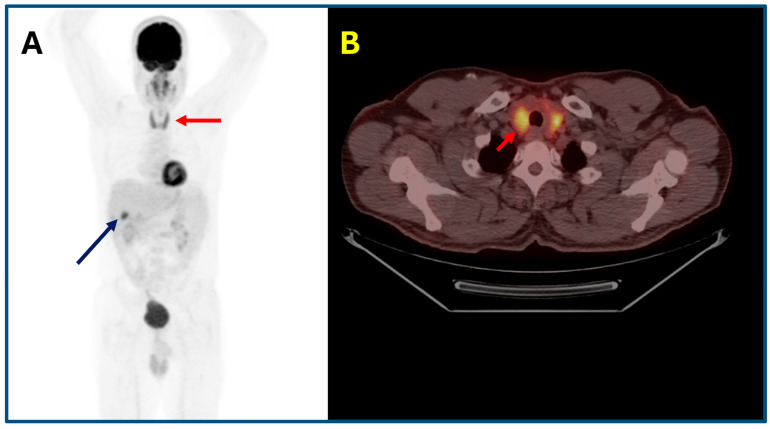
Immune-related thyroiditis in a 67-year-old male patient with colorectal cancer receiving Pembrolizumab at a dose of 2 mg/kg IV every 3 weeks for metastatic disease noted in the liver. PET maximum intensity projection (MIP) (A) and axial PET/CT fusion images (B) showed a diffuse increase in ^18^F-FDG uptake within the thyroid gland (red arrow) and ^18^F-FDG active liver metastasis (dark blue arrow).
